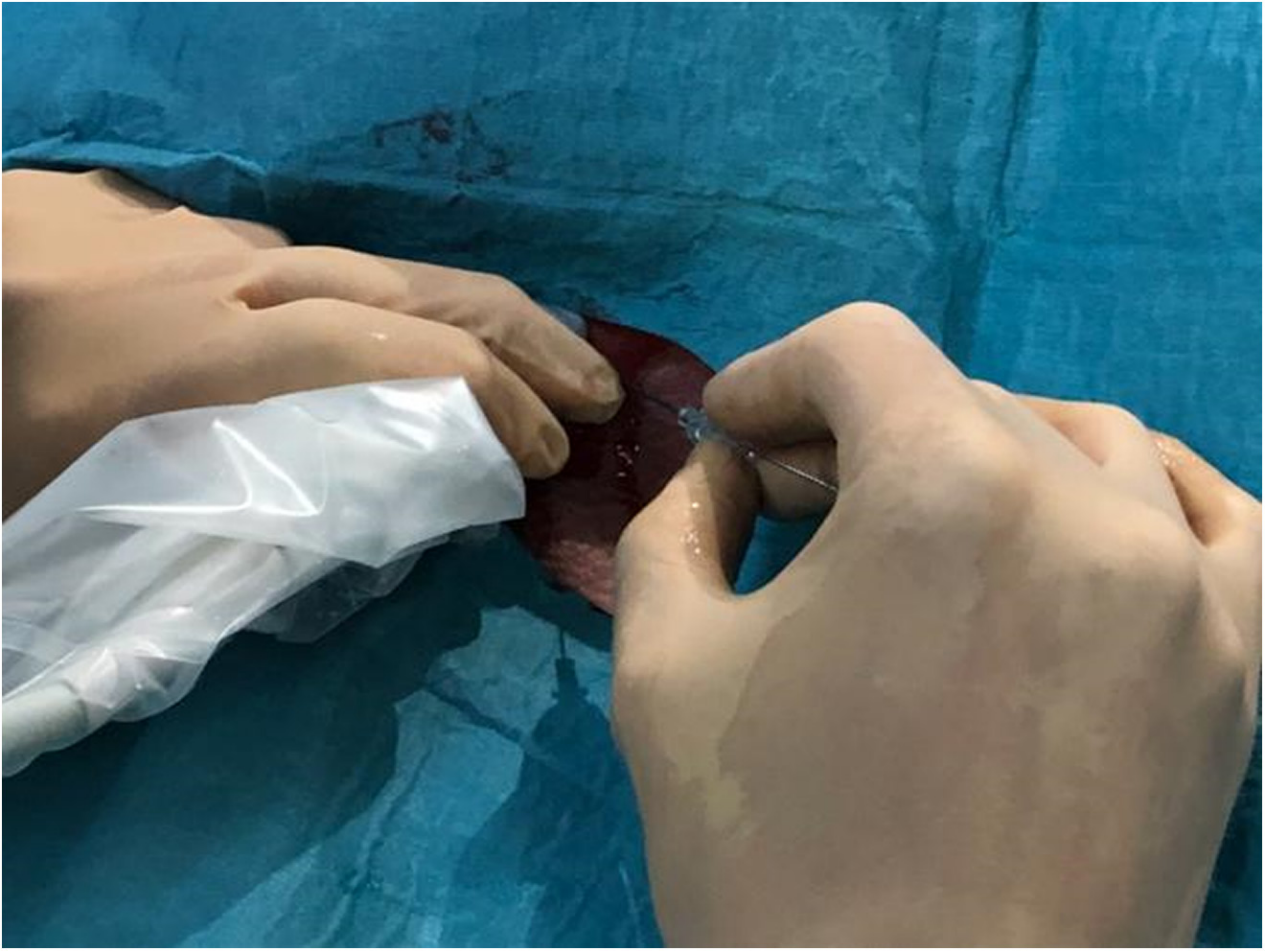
Introduction of the hook‐wire under ultrasound.
